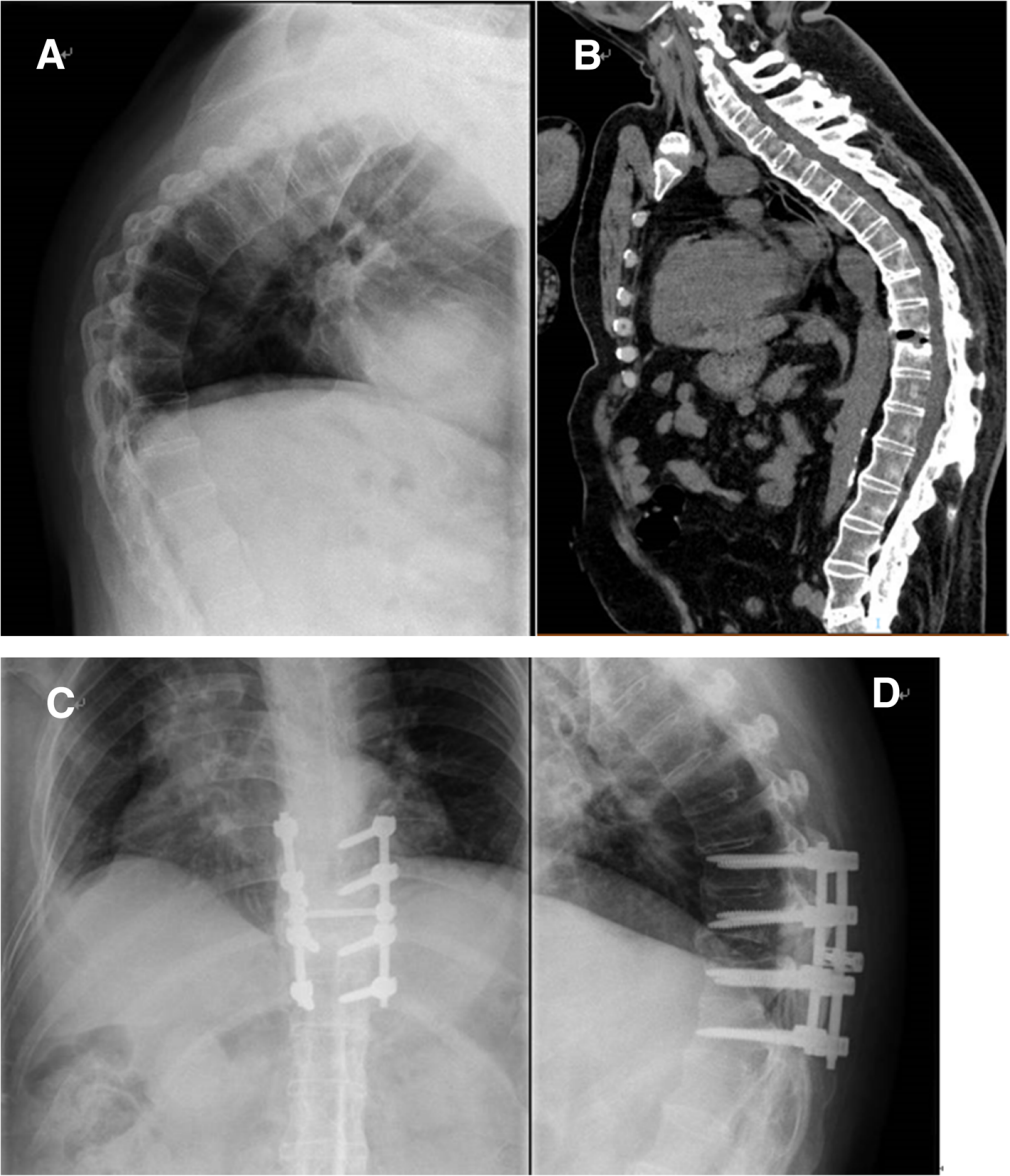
This patient had a history of AS for about 38 years, with severe kyphosis. He was admitted to the hospital due to back pain, without any obvious trauma. There was no spinal cord injury before surgery. a Preoperative X-ray shows an obvious severe kyphosis. b Preoperative CT shows obvious vertebral calcification and the fracture position is T10/11. c-d Postoperative X-ray shows satisfactory pedicle screw position and good resetting effect
